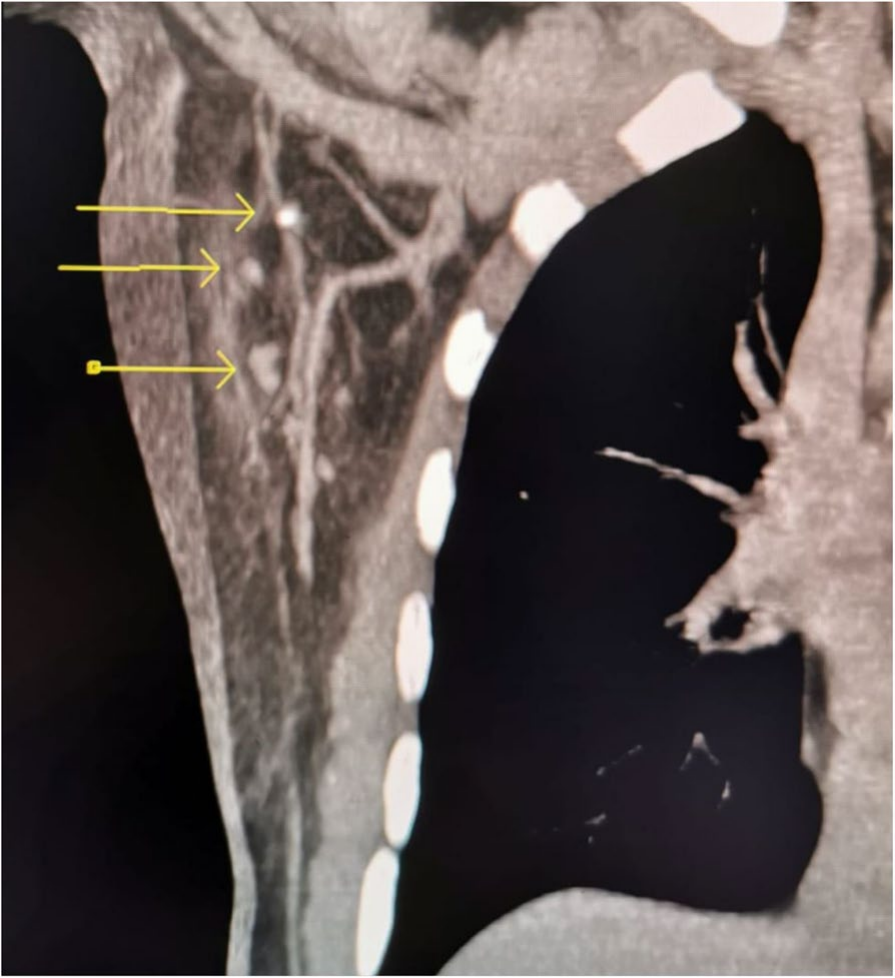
The upper arrow shows the marked lymph node with a clip inside, whereas the lower arrows indicate the other lymph nodes visible under computed tomography. The clipped lymph node was removed with ROLL along with the suspicious lymph node indicated with the lower arrow below the other lymph nodes
